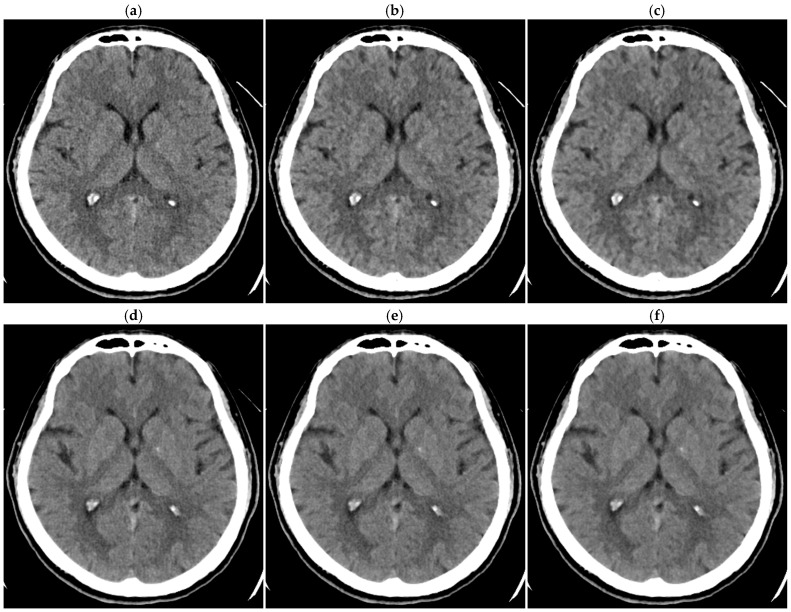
Brain CT images of 1.25 mm thickness ((a) DLR-M; (b) HIR-L; (c) HIR-H) and 5 mm thickness ((d) DLR-M; (e) HIR-L; (f) HIR-H) in a 38-year-old male patient.
